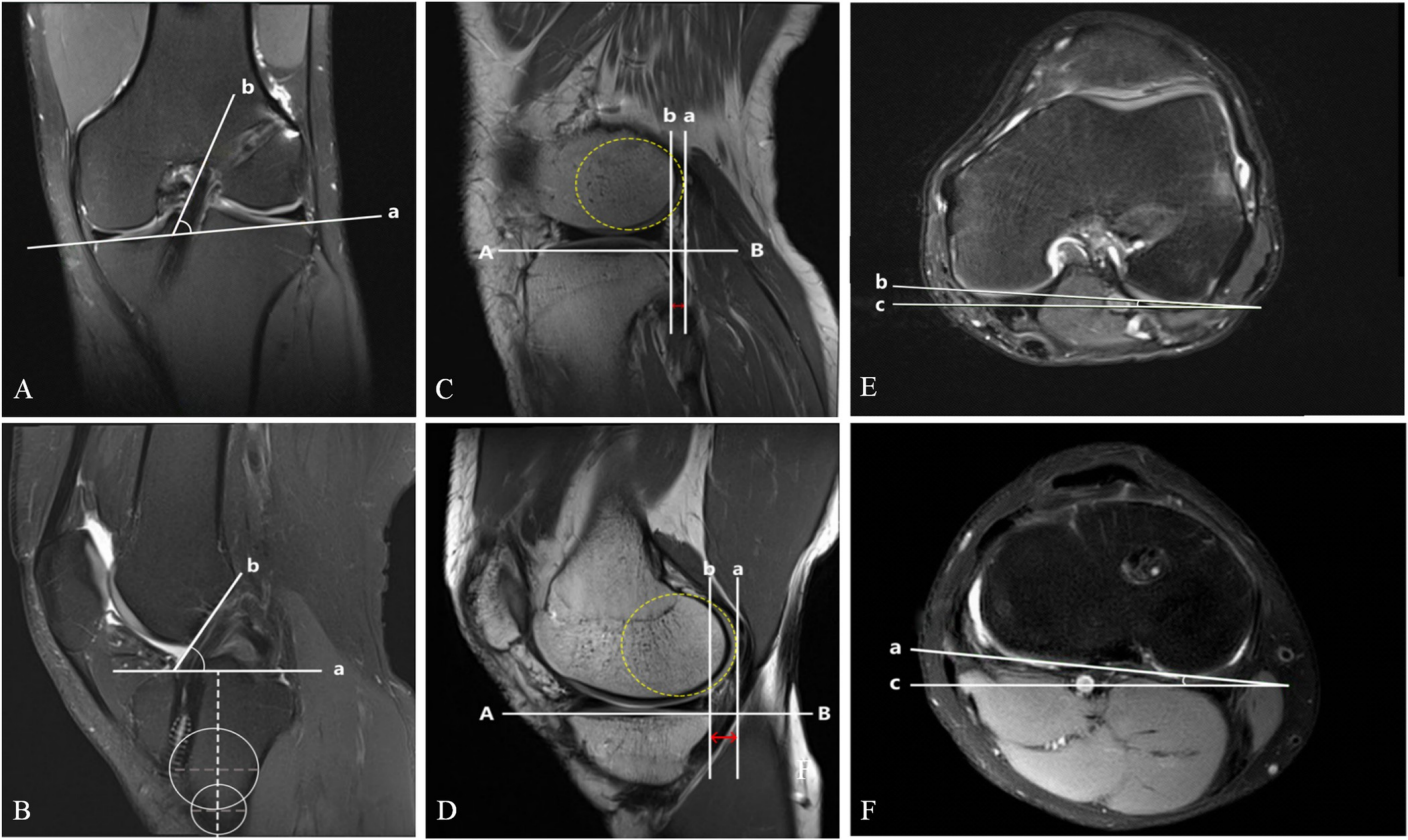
Measuring methods of various indicators based on MRI. Take the left knee joint as an example. (A) Coronal plane inclination angle; (B) Sagittal plane inclination angle; (C) Lateral ATT; (D) Medial ATT; (E) FA; (F) TA.
